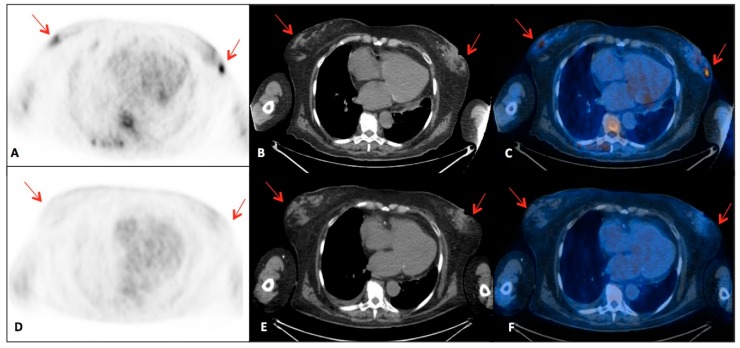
^18^F-FDG-PET/CT examinations performed in a patient affected with breast MS: axial (A,D) PET, (B,E) CT and (C,F) fusion images. (A–C) Baseline ^18^F-FDG-PET/CT shows bilateral multi-focal breast lesions with increased ^18^F-FDG uptake (SUV max 5.4) (red arrows). (D–F) Post-radiotherapy ^18^F-FDG-PET/CT demonstrates disappeared of the metabolically active lesions previously described (red arrows) and complete response to therapy.
